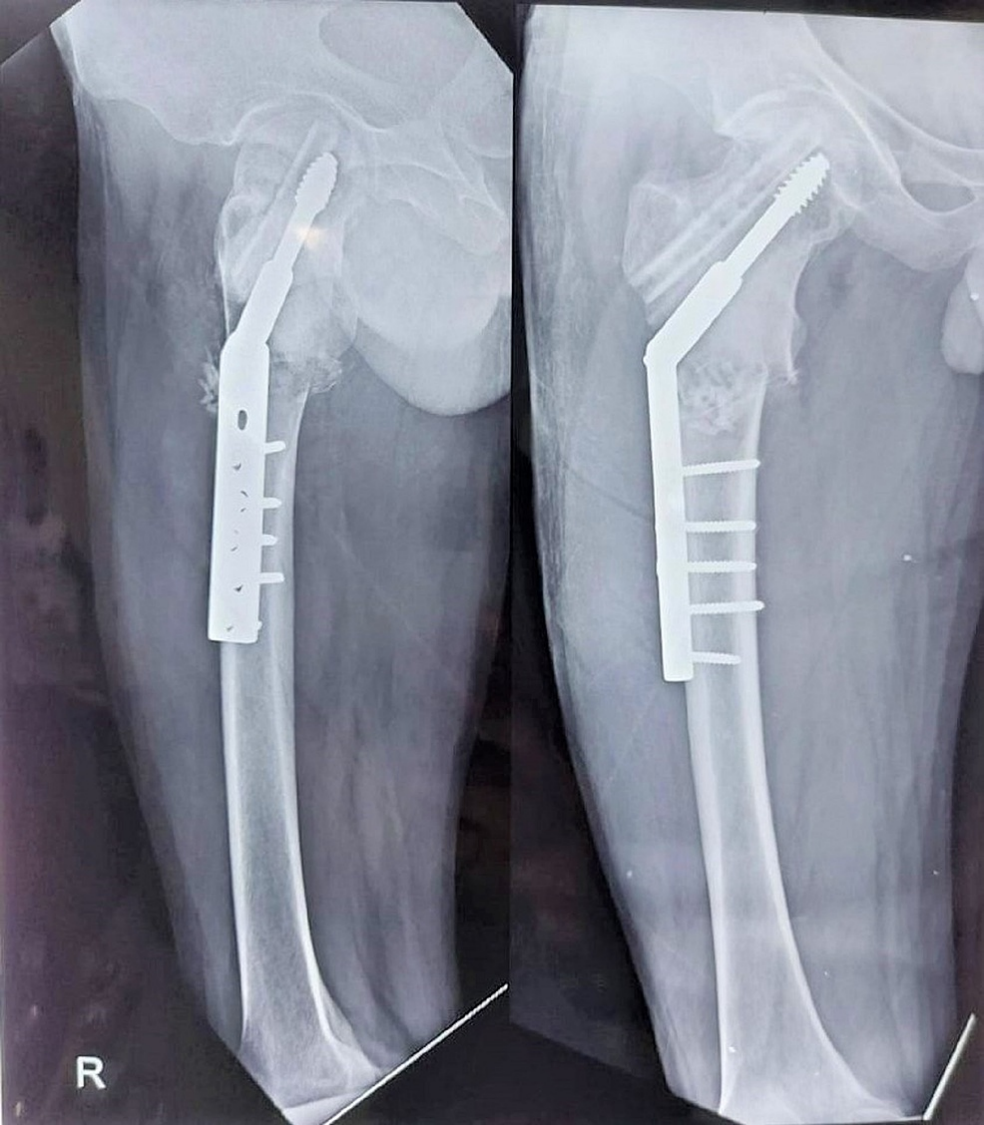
Immediate postoperative x-ray
